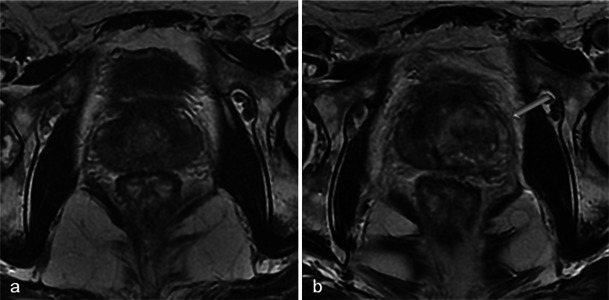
Oedema and necrosis. Left lobe photodynamic therapy in a 65-year-old patient with a PSA level of 6.2 ng/ml and two positive biopsies in the left lobe—Gleason 6 (3 + 3) adenocarcinoma. a Pre-treatment axial T2-weighted image shows diffuse bilateral hyposignal of the peripheral prostate without focal nodularity. b Day-7 post-treatment axial T2-weighted image shows a heterogeneous signal of the treated lobe extending to the peripheral and transitional zone (*arrowhead*). Note that the signal of the right lobe peripheral zone is unchanged compared with the pre-treatment image
